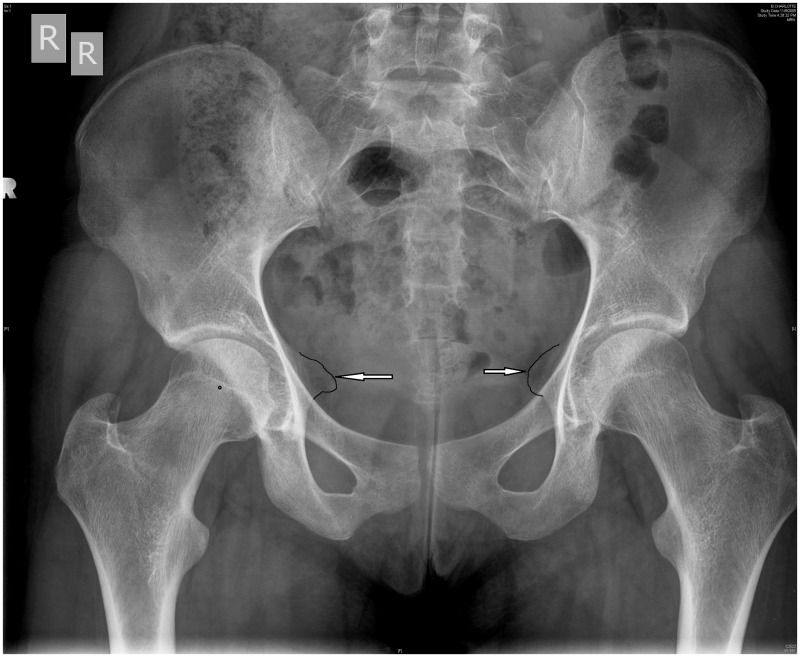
AP radiographs of a female volleyball player with right hip pain, and a history of right hip posterior dislocation. The small circle is in the center of the femoral head. Clearly, the posterior wall of the acetabulum is medial to the center of the femoral head—this is called the posterior wall sign. This demonstrates posterior wall insufficiency. The black line outlines the ischial spines with the arrows pointing to the prominent ischial spines. This would suggest the patient has acetabular retroversion, which is the reason for her posterior wall insufficiency.
